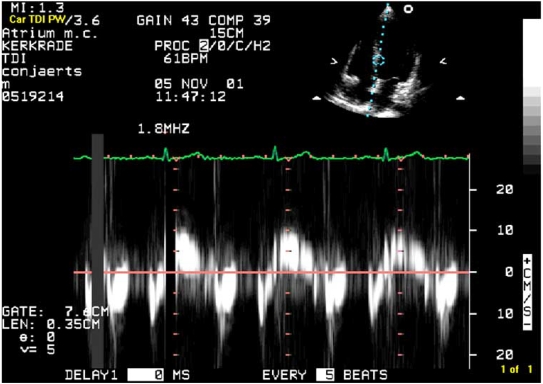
Pulsed wave Doppler at the basal septum (Fig. 2). The first positive wave is the systolic wave (Vs) the first negative wave, the early diastolic wave (Ve) and the second negative wave the late diastolic wave (Va).
